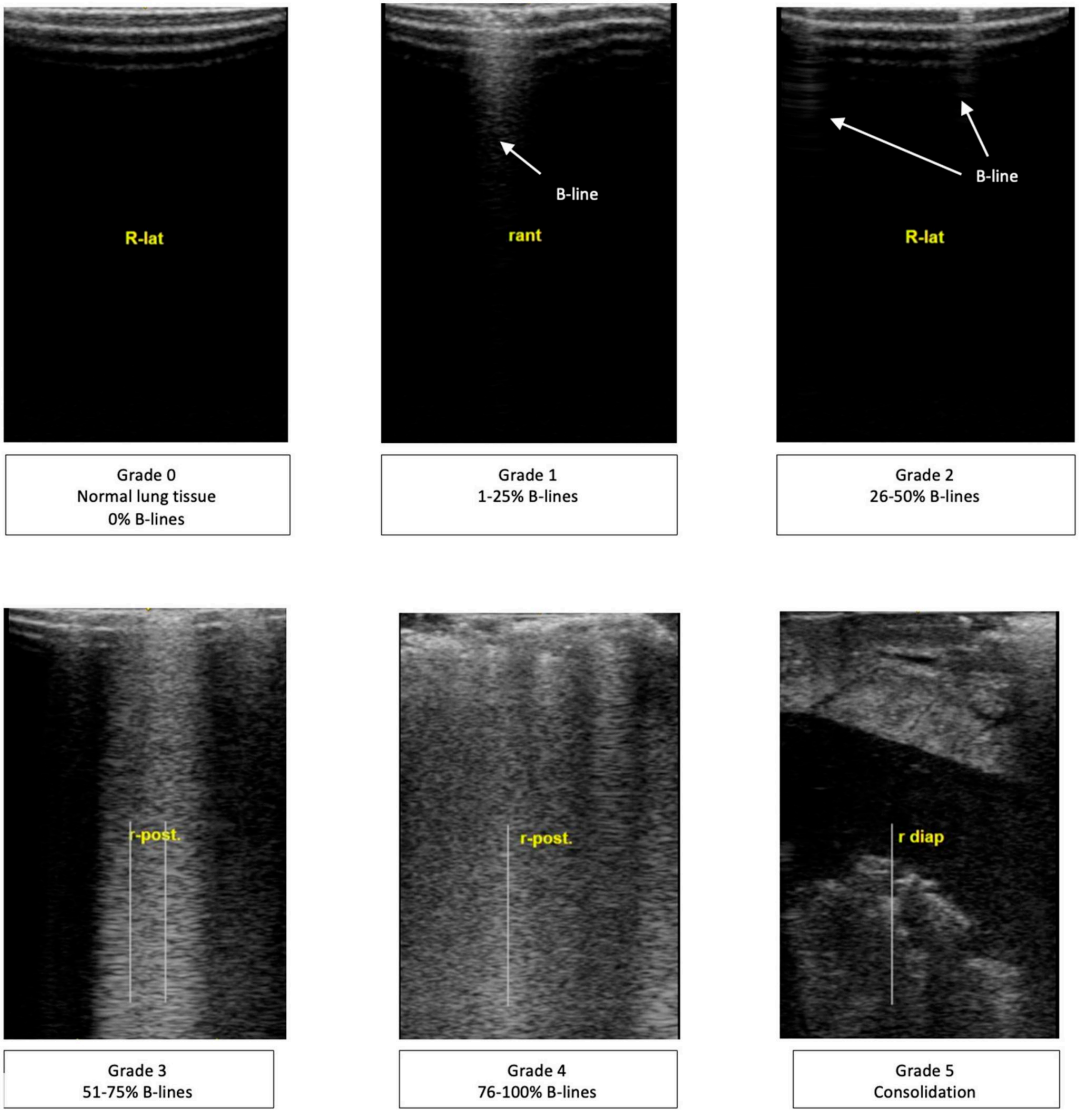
CLUE images for each grade. ant.: anterior; CLUE: DireCt Lung Ultrasound Evaluation; diap: diaphragm surface; lat.: lateral; post.: posterior; R: right lung.
